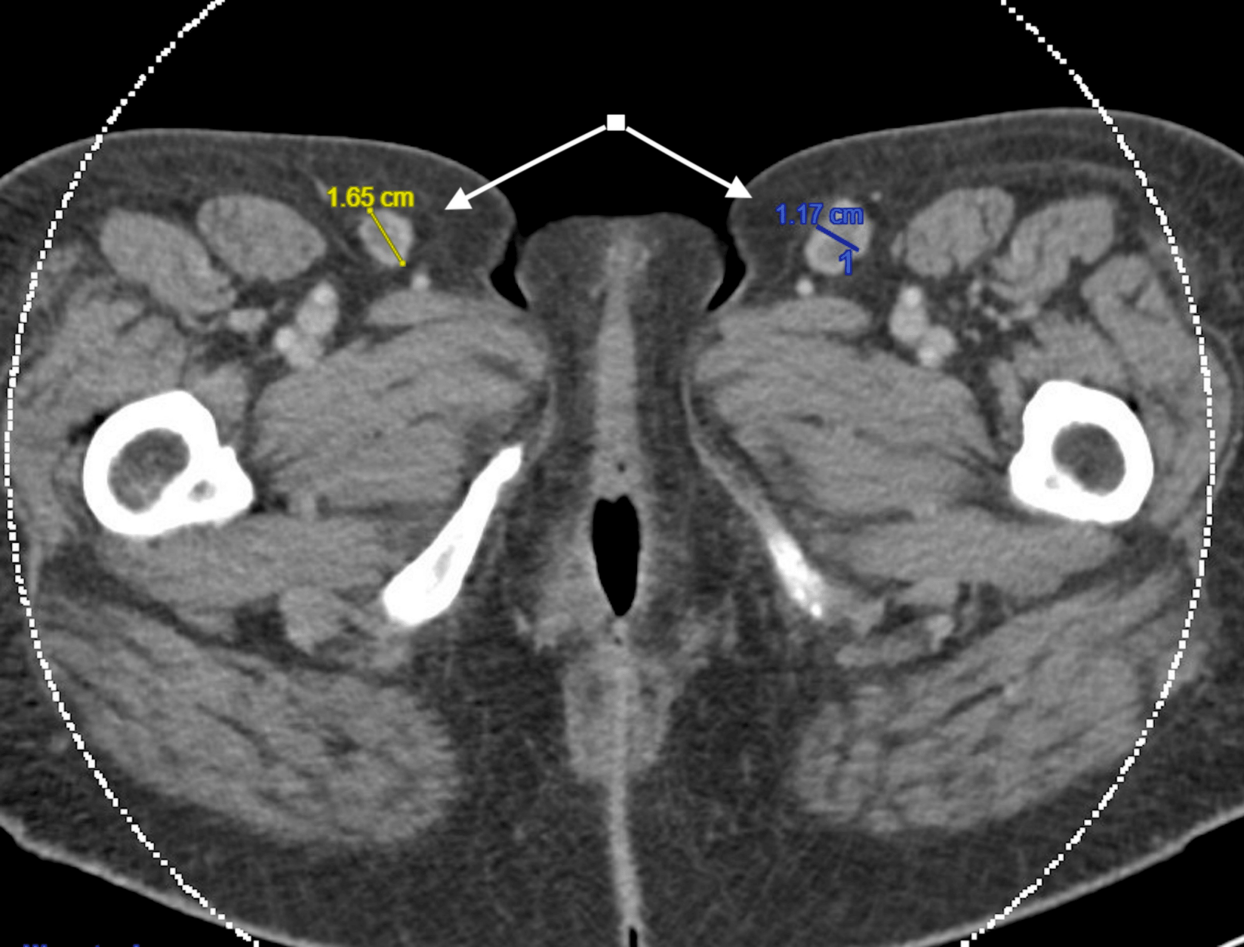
CT Scan Showing Inguinal Lymphadenopathy CT abdomen/pelvis revealed mild lymphadenopathy, most notable in the inguinal region. Lymphadenopathy qualifies as organomegaly, which is one of the minor criteria for POEMS syndrome. Other minor criteria include extravascular volume overload, endocrinopathy, skin changes, papilledema, and thrombocytosis or polycythemia. CT: computed tomography; POEMS: polyneuropathy, organomegaly, endocrinopathy, monoclonal gammopathy, and skin changes.
